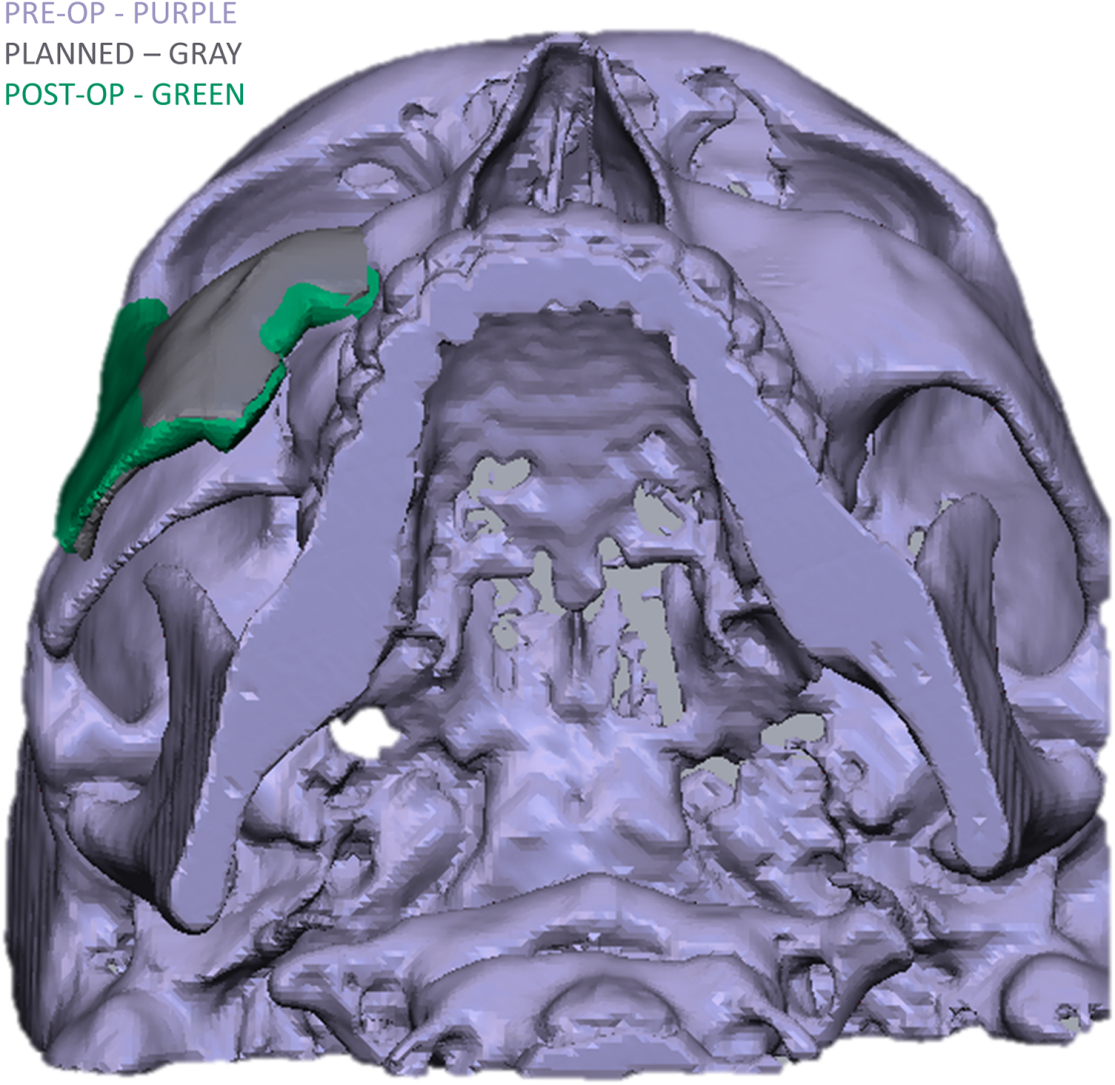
Comparison of the 3D reconstructed zygoma position in the pre, planned and post-op CT's.
